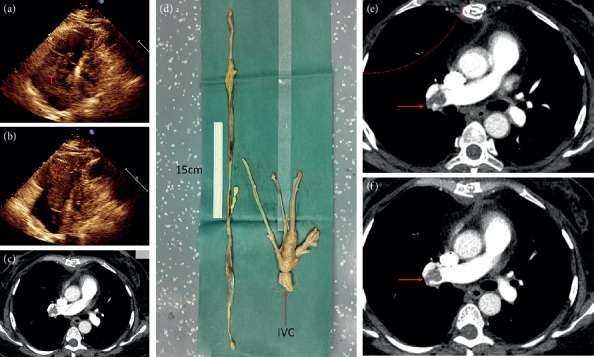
First-stage surgery for case 5. (a) Cardiac ultrasound shows systolic tumor located in the right atrium. (b) Diastolic tumor into the right ventricle. (c) Right pulmonary embolism. (d) Right atrium, right ventricle, and pulmonary artery tumor. Arrow refers to the cut off site of inferior vena cava and the length of the tumor in the heart is more than 70 cm. (e, f) CT reexamination at 3 and 6 months after surgery showed no change in right pulmonary embolism compared with that before surgery.
